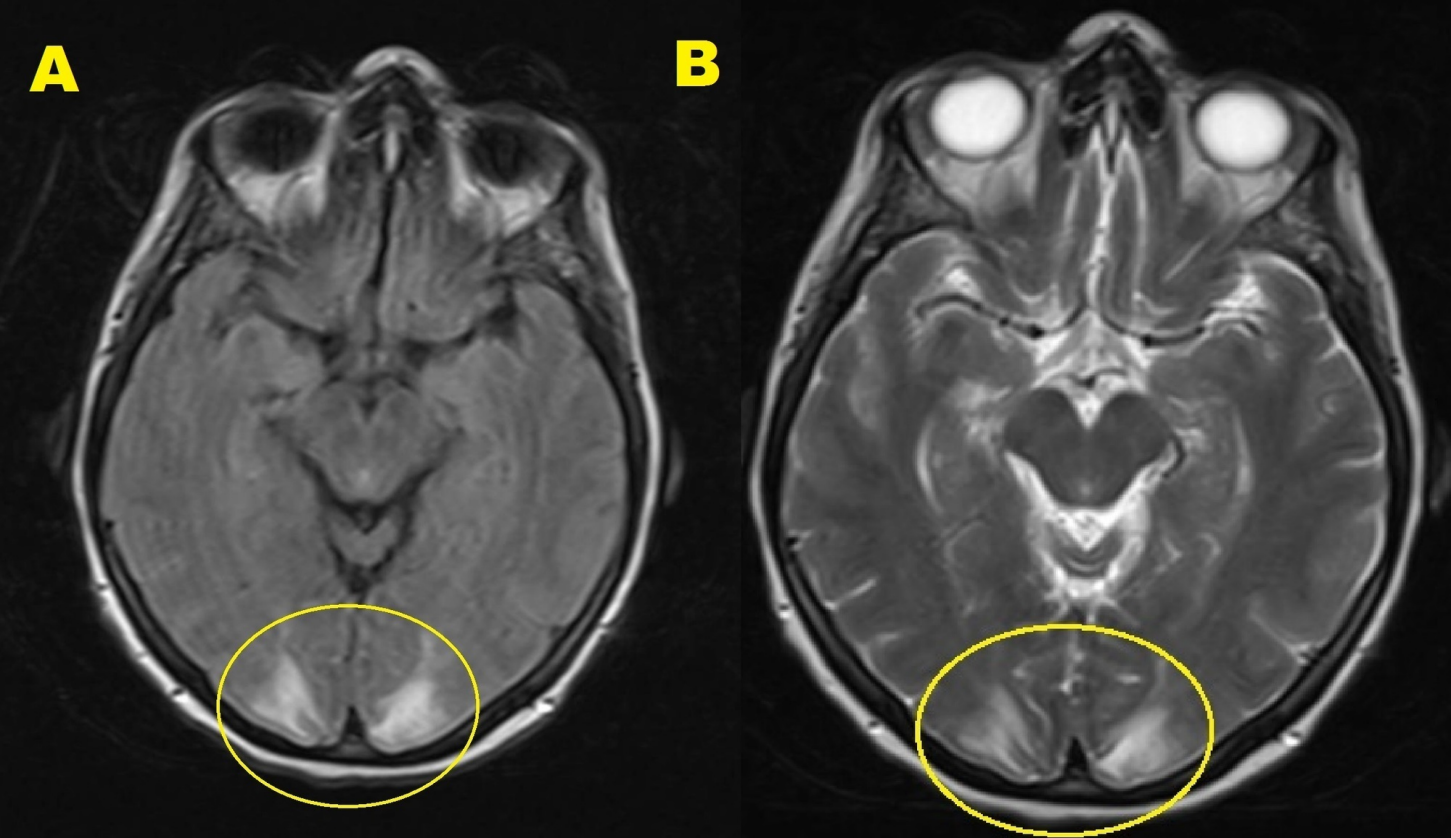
Brain MRI w/wo contrast. A) T2-TSE sequence, B) T2-FLAIR sequence. Showing bilateral, symmetric areas of T2-FLAIR hyperintensity involving the posterior parietal and occipital lobes. There was no evidence of acute stroke, intracranial hemorrhage, hydrocephalus, dural venous sinuses thrombosis, or intracranial metastatic disease. T2-TSE: T2-weighted turbo spin echo; T2-FLAIR: T2-weighted-fluid-attenuated inversion recovery.
